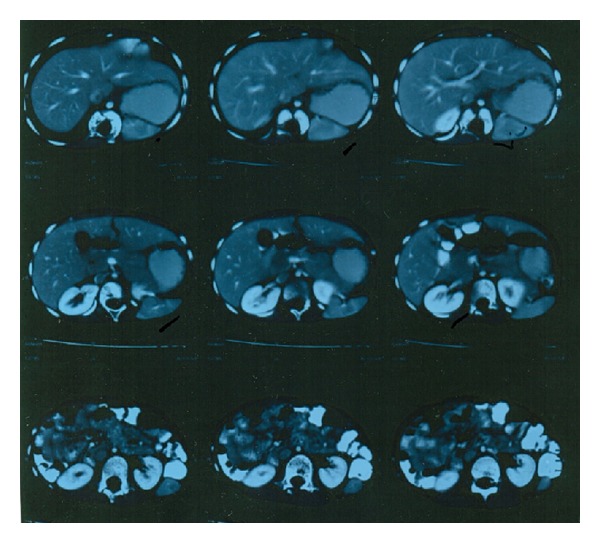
CT scan showing severely hypoplastic posterior abdominal muscles (erector spinae, iliocostalis, and latissimus dorsi) causing posterolateral herniation of the spleen. D10–12 vertebral bodies are hemivertebrae.
